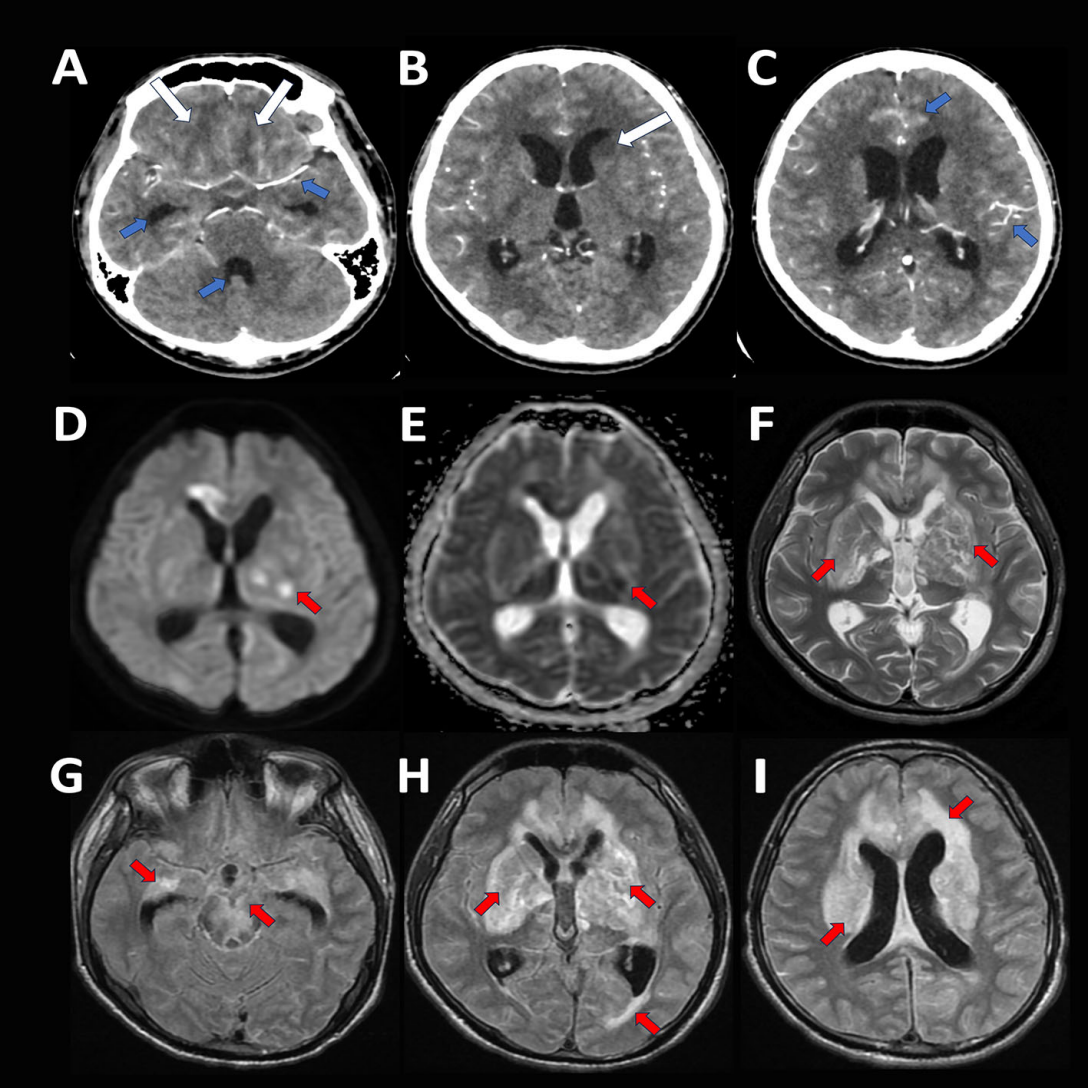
Neuroimaging of the patient. Cranial computed tomography scan with contrast (A-C): Fairly defined focal areas of hypodensities seen in the deep white matter of the bilateral basofrontal parasagittal lobes, head of the left caudate nucleus, and anterior limb of the left internal capsule (A,B: white arrows), with undue leptomeningeal enhancement and mild communicating hydrocephalus (A,C: blue arrows). Cranial MRI with contrast (D-I): Hyperintense foci with some areas of restricted diffusion and magnetic susceptibility artifacts with surrounding vasogenic edema in the cortical-subcortical junction of the bilateral basal ganglia, thalamus, midbrain and pons, bilateral temporal, bilateral parietal, left occipital lobes and bilateral frontal lobes (D-I: red arrows); with leptomeningeal and pachymeningeal nodular thickening and enhancement.
